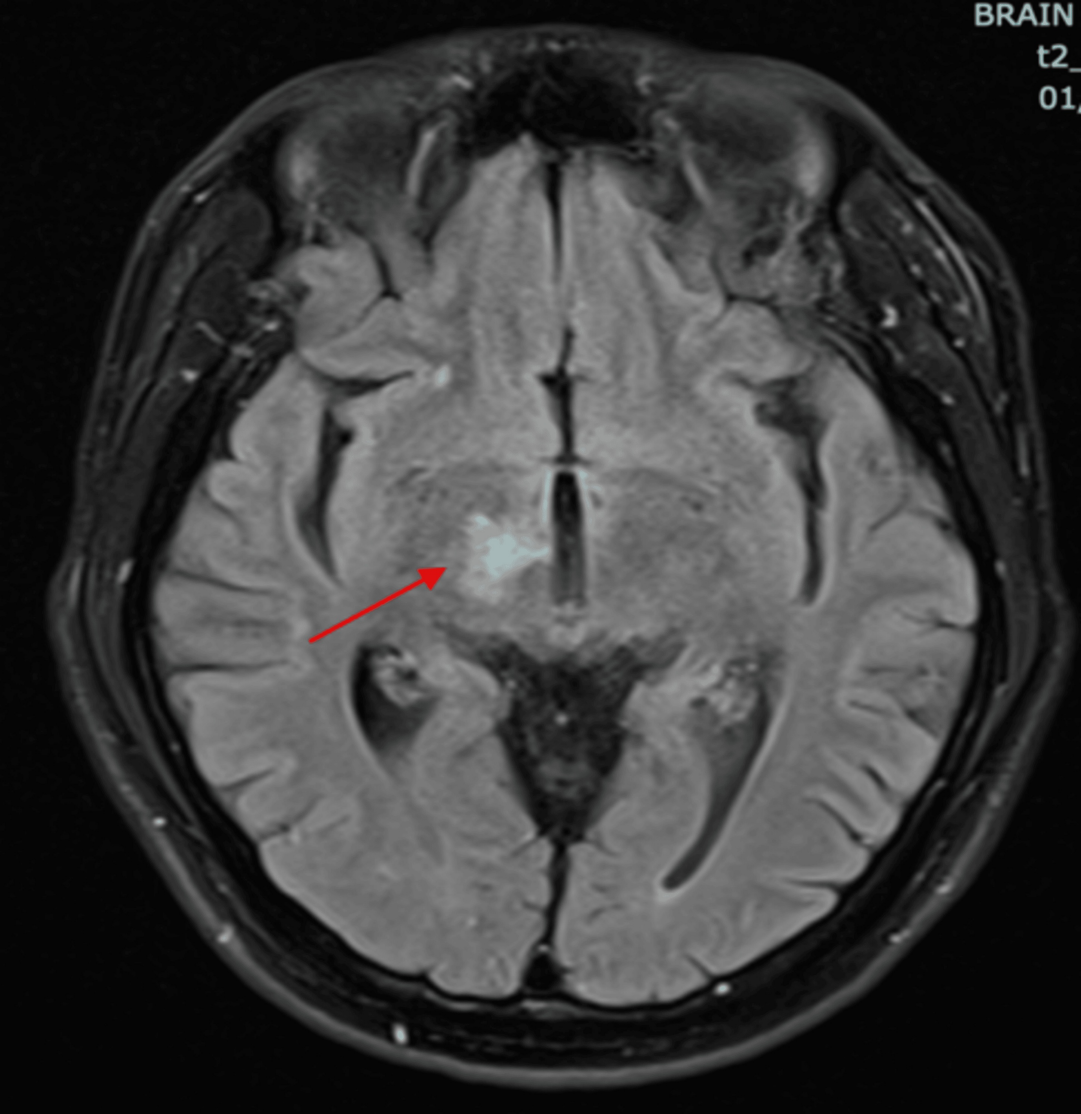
MRI of the brain T2 FLAIR showed hyperintensity at the right thalamus with perilesional edema (thin red arrow). T2-FLAIR: T2-weighted-Fluid-Attenuated Inversion Recovery
